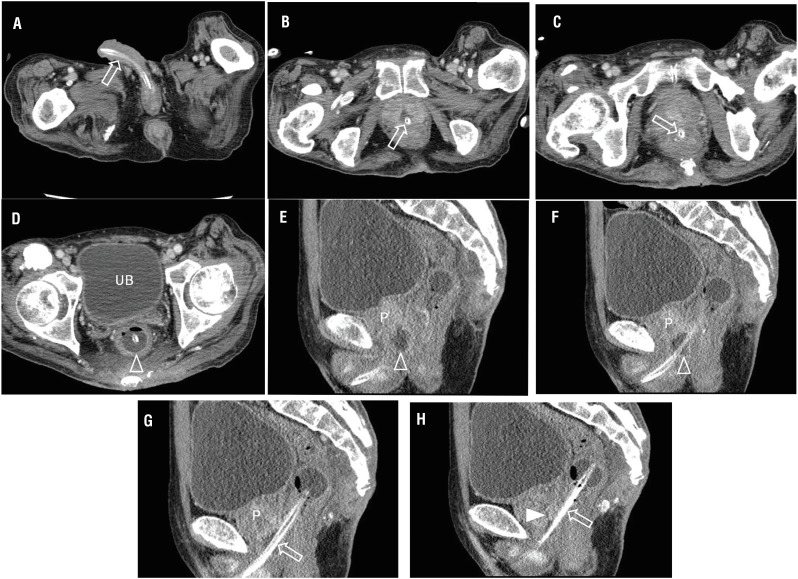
(A-D) Serial axial images of abdominal computed tomography shows malposition of the Foley catheter. It is located along the urethra (arrows, A) -prostate (arrow, B) -rectum (arrow, C), indicating presence of the recto-urethral fistula. The balloon (arrowhead, D) of the Foley catheter is located in the rectum, and not in the urinary bladder (UB). (E-H) Serial sagittal images of abdominal computed tomography reveals the penetration (arrows, G and H) of the prostate (P) with loculated fluid collection with air bubbles in the postero-inferior aspect of the prostate (open arrowhead, E and F), indicating a prostatic abscess. This abscess is bulging and abutting to the anterior wall of the rectum. Normal prostatic urethra is also seen (arrowhead, H). Additionally, there is soft tissue tumefaction involving the presacral space, indicating inflammation. However, bone window setting did not demonstrate bone involvement (no evidence of osteomyelitis).
